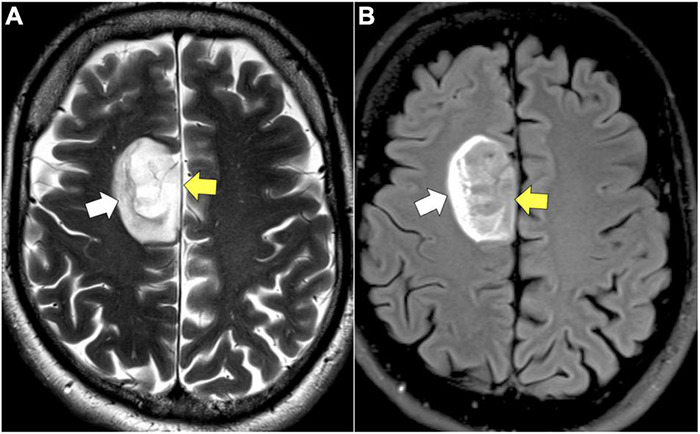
T2/FLAIR mismatch. (A) Axial T2: homogenously hyperintense mass (yellow arrow). (B) Axial FLAIR: hypointense mass (yellow arrow) relative to T2 image with exception of a hyperintense peripheral rim (white arrow).
